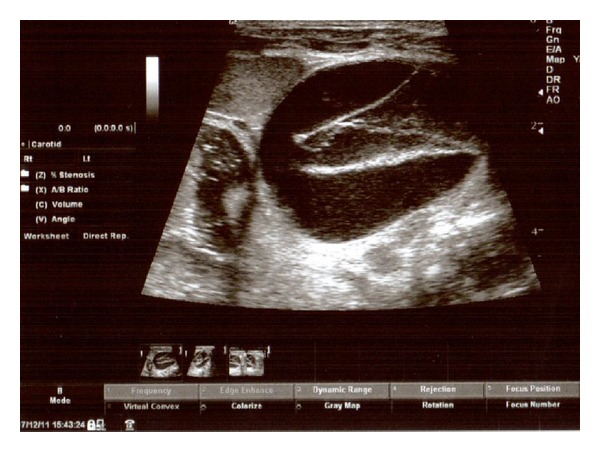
Postnatal ultrasonographic appearance with marked septations.
